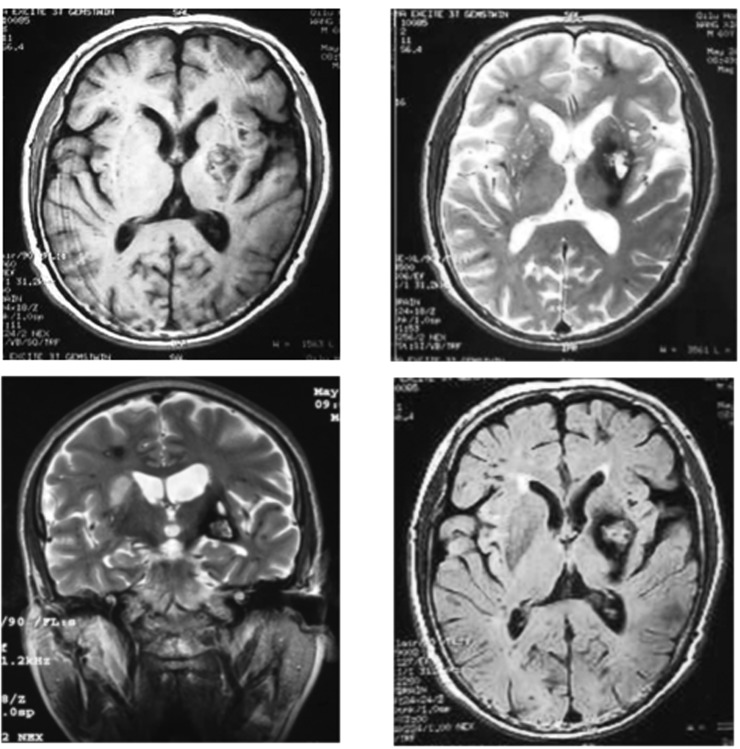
(A, B and C) Foci of CCM show long T_1_ and T_2_ signal intensity in conventional MRI scans and high signal intensity in DWI scans with a distinct boundary. (A and B) The large focus typically has mixed T_1_- signal intensity with a core of mixed high and low T_2_-signal intensity surrounded by a low signal intensity, and a distinct boundary. (D) These lesions demonstrated high signal intensities surrounded by a low signal intensity on T_2_Flair images. CCM, cerebral cavernous malformation; MRI, magnetic resonance imaging; DWI, diffusion-weighted imaging.
